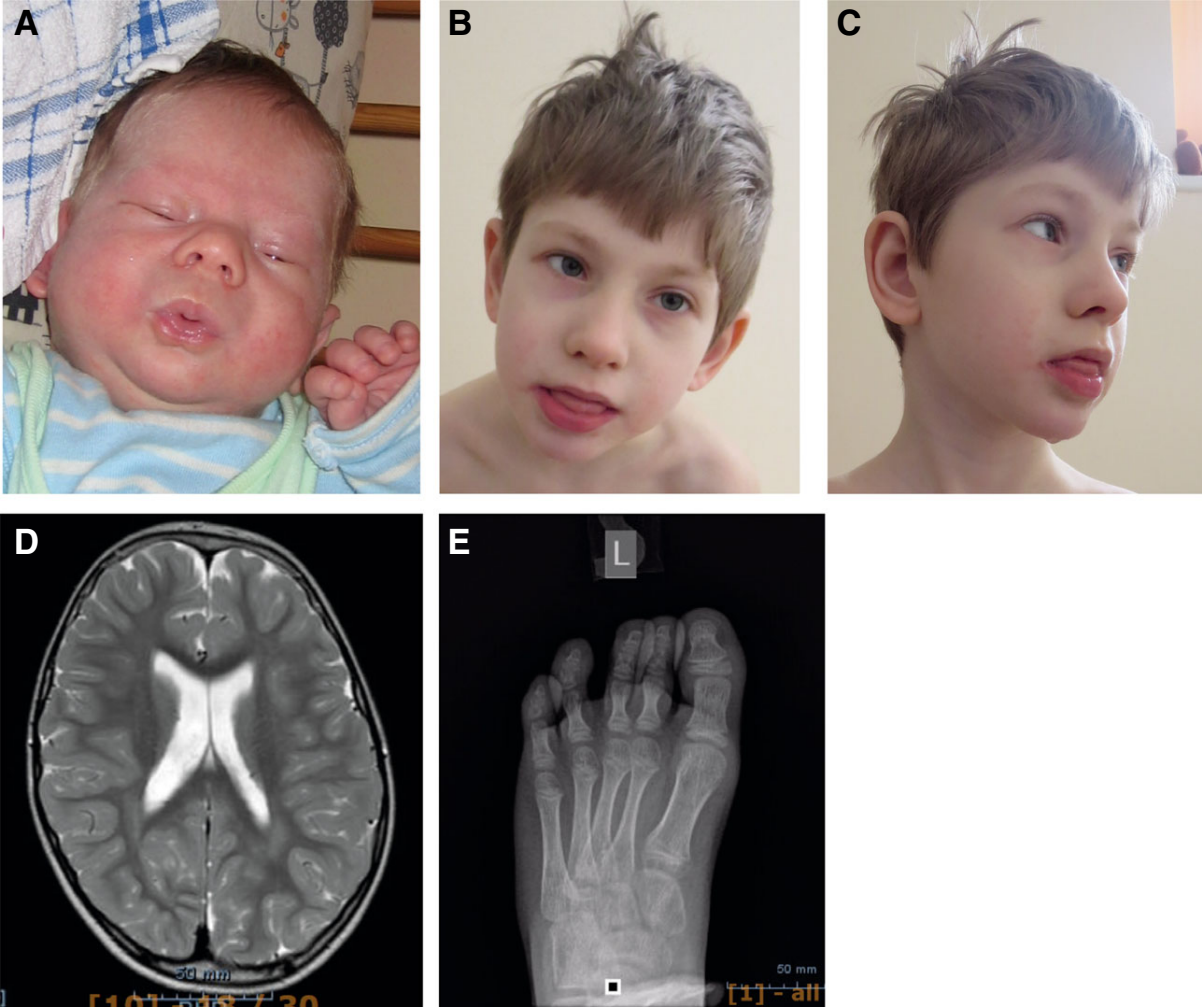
Clinical pictures from patient carrying the N648Y mutation in the catalytic domain of OGT. (A) Facial asymmetry at birth. (B) Facial view at 7 years. (C) Profile view at 7 years. Note coarse facial features, drooling. (D) MRI image showing brain atrophy and mega cisterna magna. (E) X‐ray image of the left foot—cone‐shape epiphyses.
